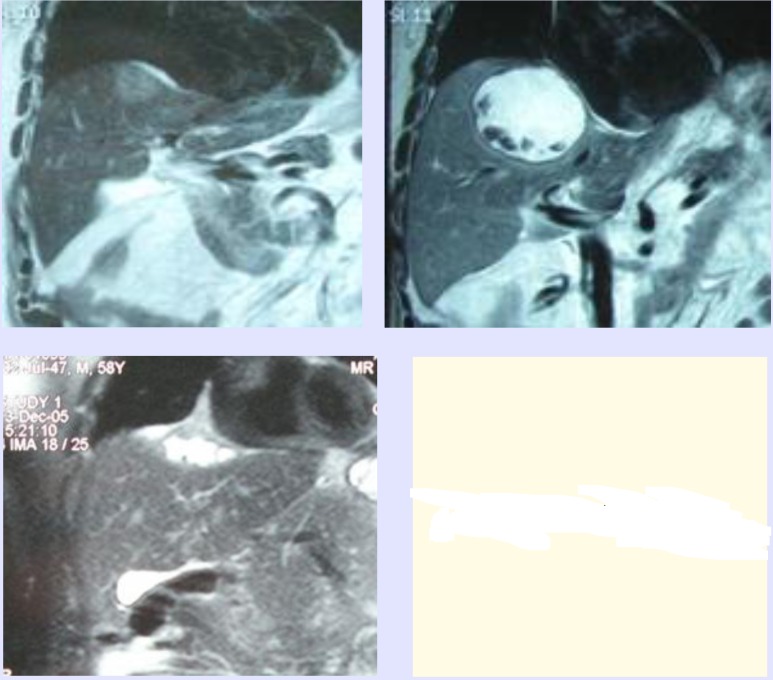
MRI of liver: MKRC before, immediately after RFAR and after 8 months
